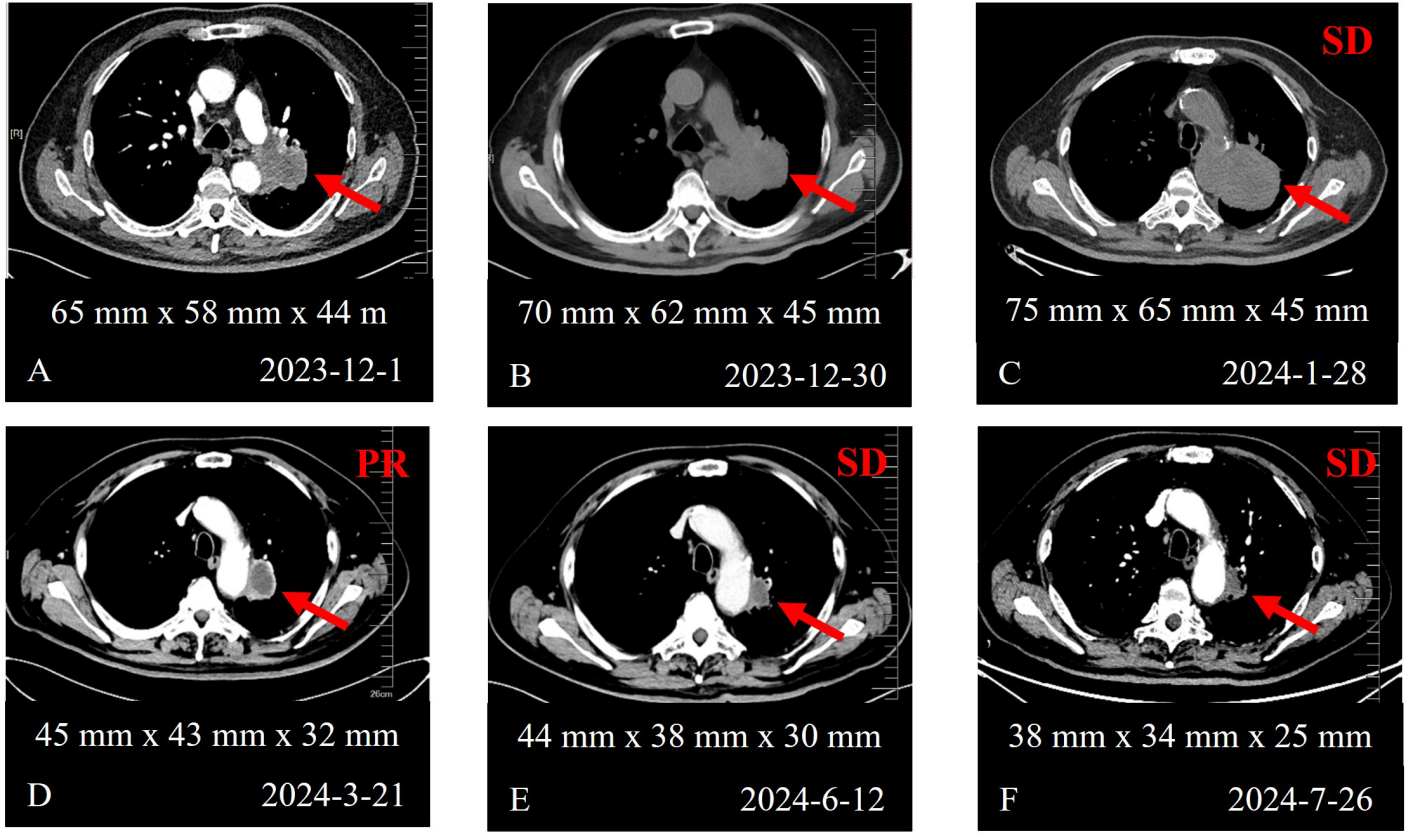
Chest CT images demonstrating the evolution of the primary lung lesion before and after treatment. (A) Baseline scan at initial diagnosis. (B) Scan before treatment initiation after definitive diagnosis. (C) Scan after 1 cycle of toripalimab, pemetrexed, and carboplatin. (D) Scan after 1 cycle of toripalimab, pemetrexed, and carboplatin, followed by 2 subsequent cycles of sotorasib combination therapy. (E) Scan after 2 cycles of sotorasib plus toripalimab combined with pemetrexed and carboplatin, and 1 additional cycle of sotorasib plus toripalimab. (F) Scan after 2 cycles of sotorasib plus toripalimab therapy.
